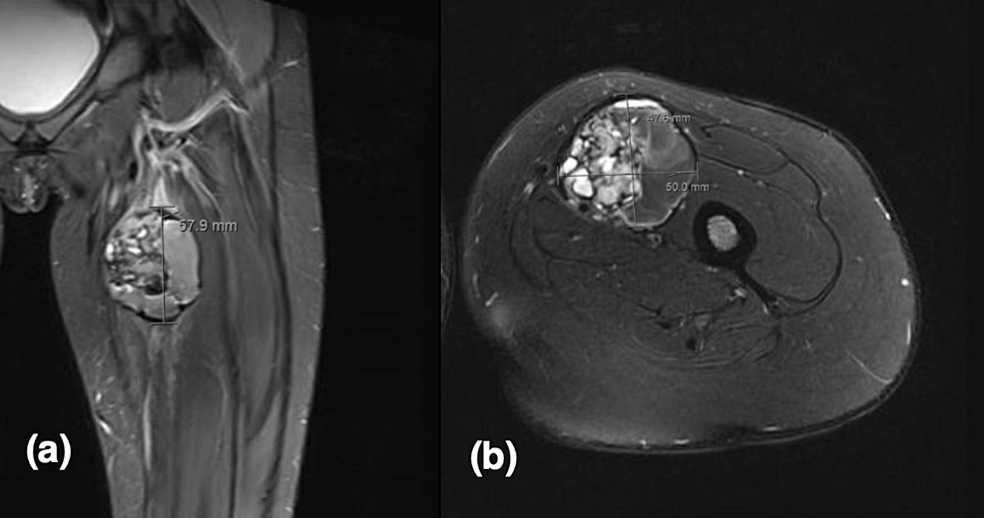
A 12-year-old boy diagnosed with angiomatoid fibrous histiocytoma (case 2): (a) Coronal and (b) axial T2-weighted fat-saturated images. A 5.8x4.8x5.0 cm oval mass showing in the proximal left thigh.
